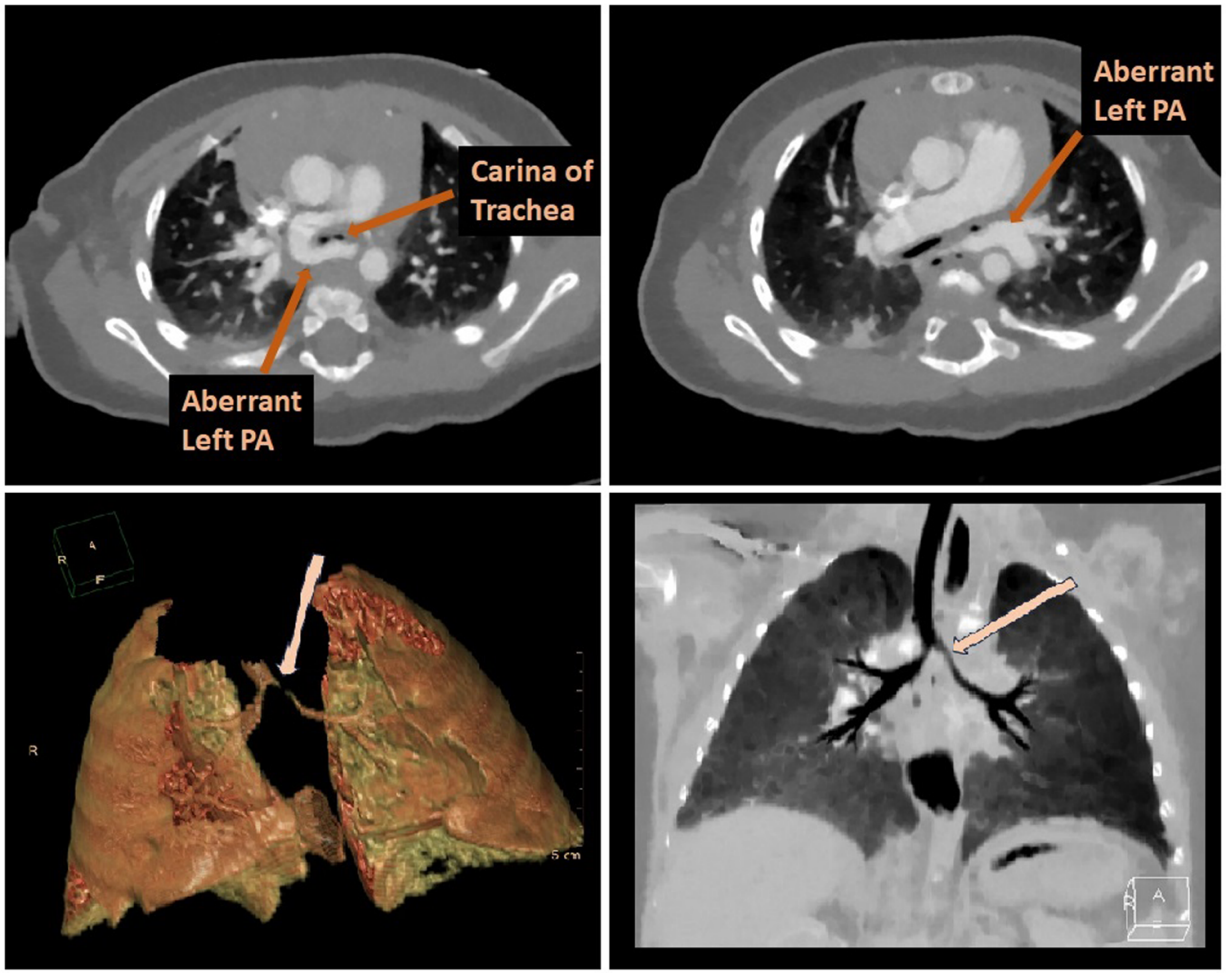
The CT scan confirmed that the left pulmonary artery was originating from the right pulmonary artery and coursing behind the trachea, leading to tracheal compression.
